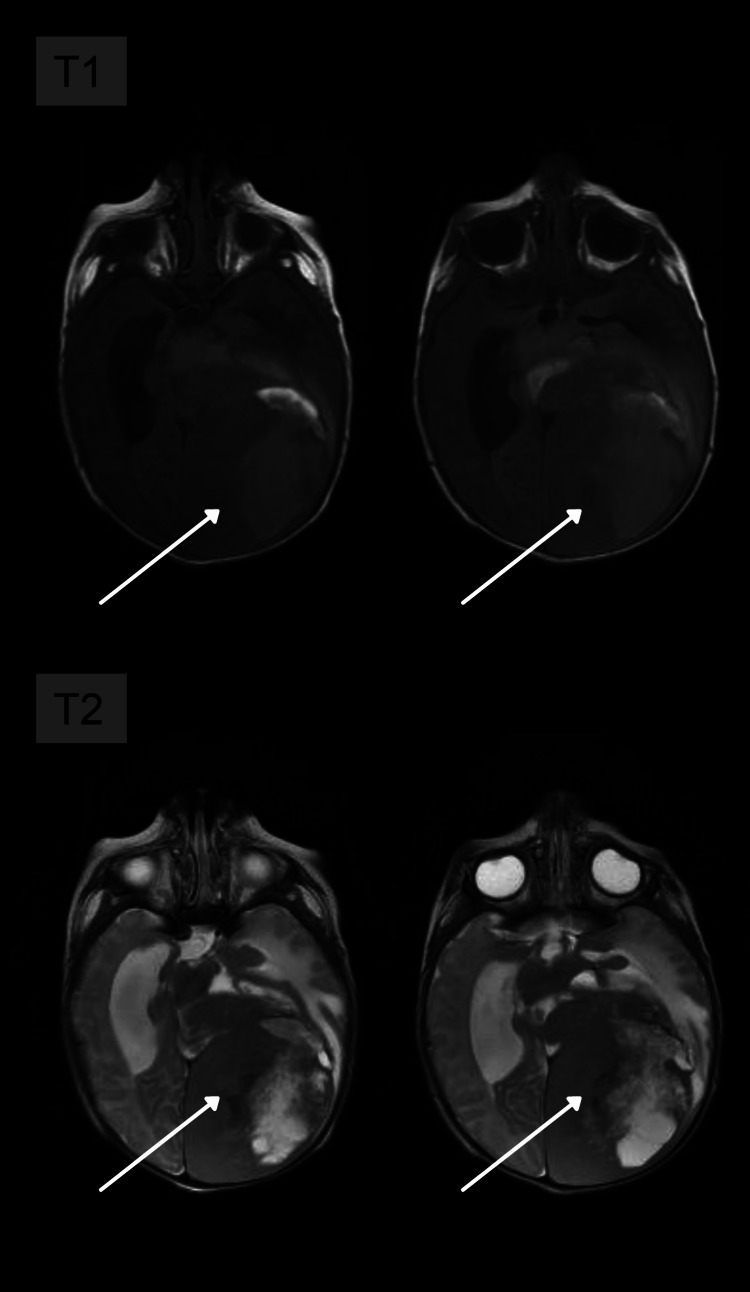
T1-weighted axial images showing a large expansive hemispheric lesion on the left, temporoparietal, heterogeneous, imprecise contours, with areas of high signal, probably correlated with hemorrhagic content. T2-weighted axial images, with a very heterogeneous lesion matrix, as well as areas of the hyperintense signal that may be associated with necrosis or cystic degeneration.
